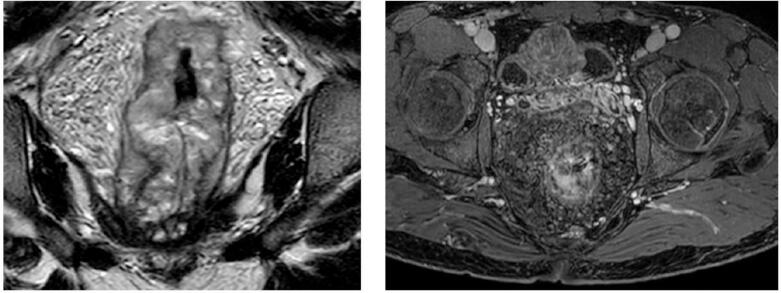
Pelvic MRI scan with marked irregular circumferential mucosal and submucosal thickening involving the anorectal wall, about 2.3 cm from the anal verge, extending into the distal part of the sigmoid colon, measuring about 1.5 cm in thickness and 16 cm in length, associated with numerous serpiginous flow void structures showing enhancement on postcontrast sequences.
